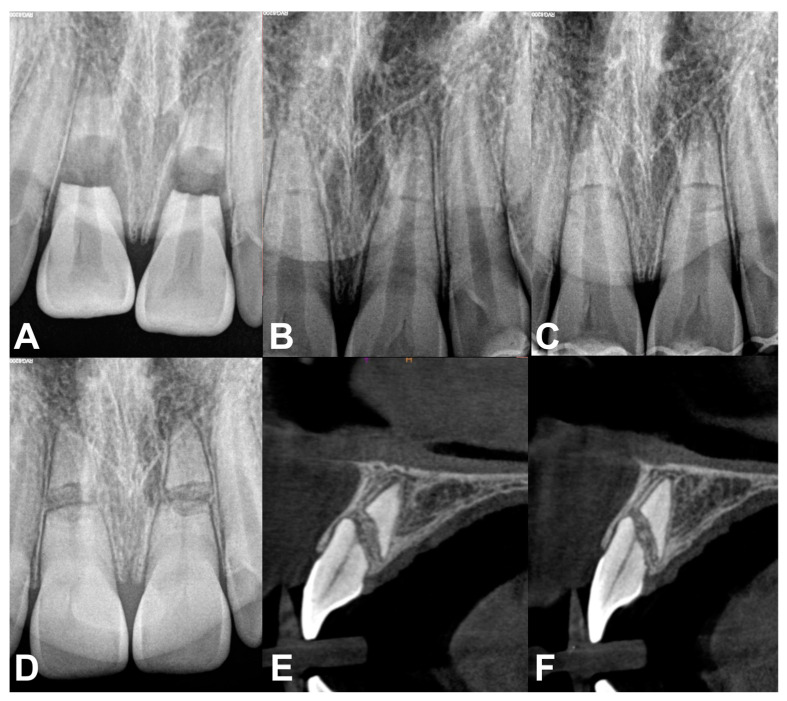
Dental radiographs and CBCT: (A) Radiograph taken on the day of the injury; (B) radiograph after repositioning and splint application; (C) radiograph taken 4 weeks after the injury; (D) one year after the injury—bony bridge separating the fragments is seen with a periodontal space around both parts and pulp canal obliteration is present; (E) CBCT of the right central maxillary incisor after 14 months post trauma; (F) CBCT of the left central maxillary incisor after 14 months post trauma.
